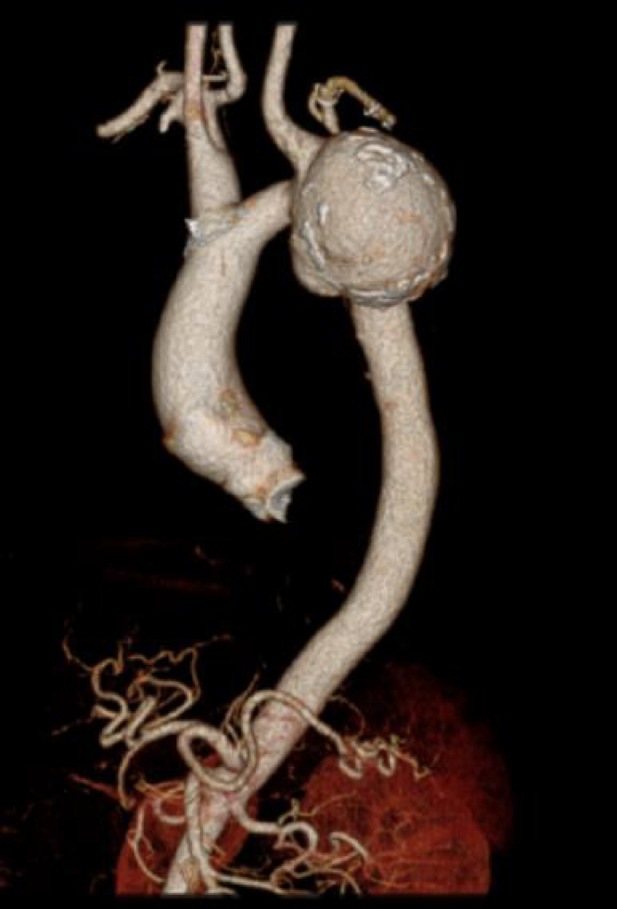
Three-dimensional CTA reconstruction demonstrates the aneurysm with the left carotid artery originating from the aneurysmal sac
